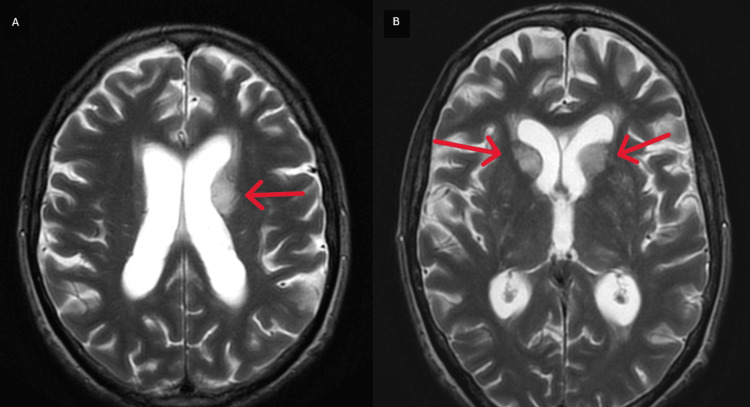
Axial view of the cerebral MRI T2 FSE showing the tumefactive lesions on the caudate nucleus bilaterally (red arrows) MRI: magnetic resonance imaging, FSE: fast spin-echo
